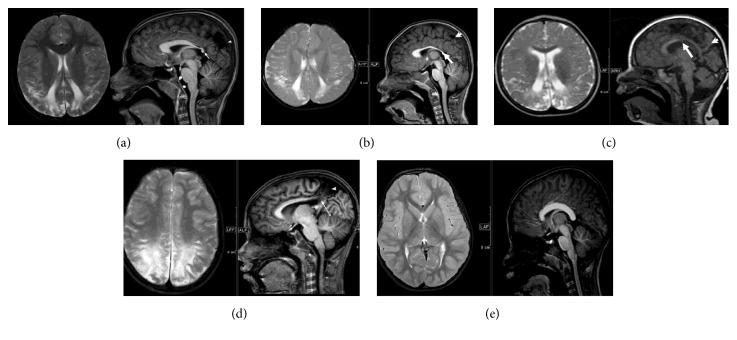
T2 axial and T1 sagittal MRI brain scans of the 5 children described. Cases (a)–(c)—bilateral symmetric parietooccipital gliosis (arrowhead) with regional white matter volume loss, thinning in the posterior body, isthmus, and splenium of the corpus callosum (white arrow) being evident. Associated white matter hyperintensities and ventricular dilatation are seen. Case (d)—bilateral symmetric parietal gliosis was seen (arrowhead) with marked thinning of the isthmus and splenium of the corpus callosum (long white arrow). Case (e)—a near normal scan with prominence of the atria and mild shortening of the corpus callosum and splenium, with thinning of the splenium.
